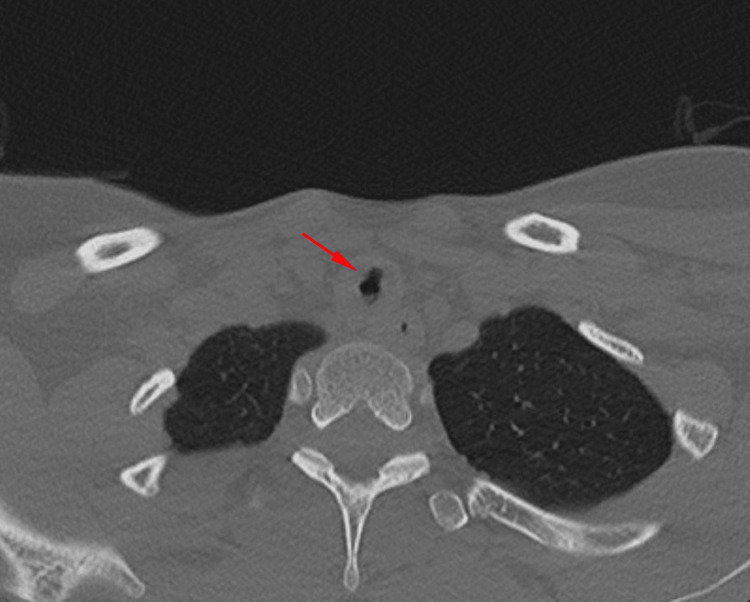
CT soft tissue neck showing 40% tracheal narrowing 6.5 cm below the laryngeal inlet
